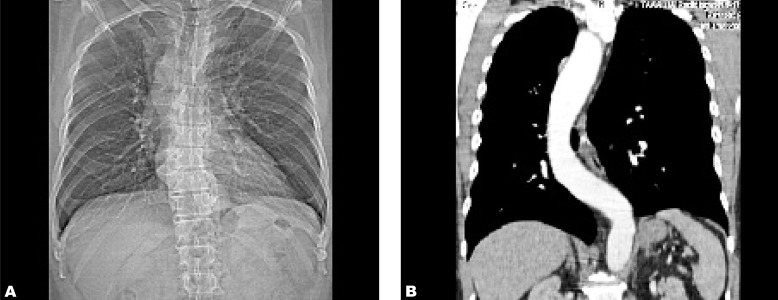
A 52-year-old man with right-sided aortic arch and aberrant left subclavian artery. A. Chest radiograph shows widening of the right mediastinum with right-sided aortic arch. B. Coronal multiplanar reformatted CT image of the right-sided thoracic aorta.
